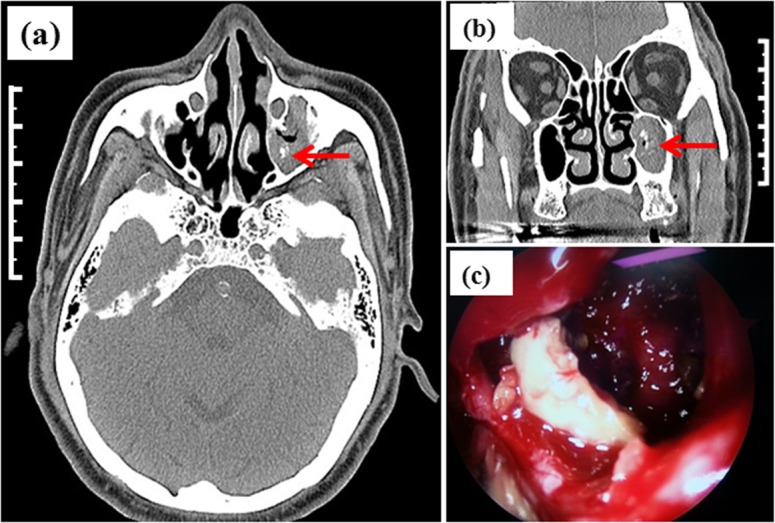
The axial (a) and coronal (b) CT image of case No.3 and the photograph of fungus ball taken through an endoscope (c) (The total length of scale bar shown in (a) and (b) is 5 cm.).
